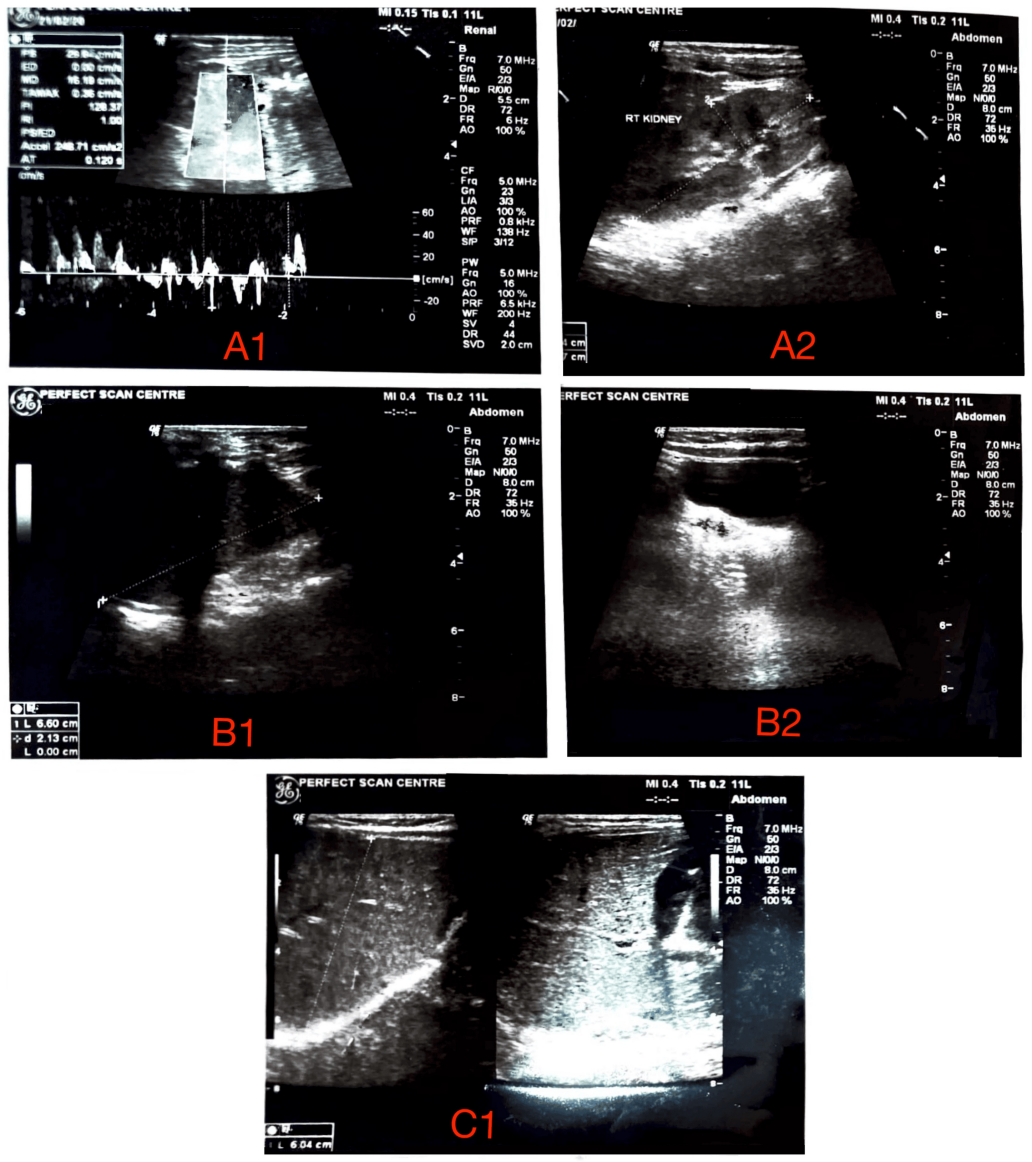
Postoperative Doppler and grayscale ultrasonographic assessment in the pediatric patient with a history of unilateral MCDK status post left nephrectomy. (A1) Renal artery spectral Doppler waveform of the right kidney demonstrating normal resistive index and acceleration time, indicating preserved vascular patency and hemodynamics. (A2) Longitudinal grayscale sonogram of the right kidney showing intact corticomedullary differentiation, homogeneous parenchymal echotexture, and normal dimensions for age, with no evidence of hydronephrosis or focal lesions. (B1) Longitudinal grayscale view of the right kidney with caliper measurements confirming appropriate renal length and cortical thickness. (B2) Transverse grayscale view of the right kidney reaffirming normal renal architecture without cystic or solid masses. (C1) Longitudinal and transverse grayscale views of the right kidney hilum displaying normal renal sinus echogenicity, intact perihilar structures, and no perinephric fluid collections, consistent with a healthy, solitary functioning kidney. MCDK, multicystic dysplastic kidney
